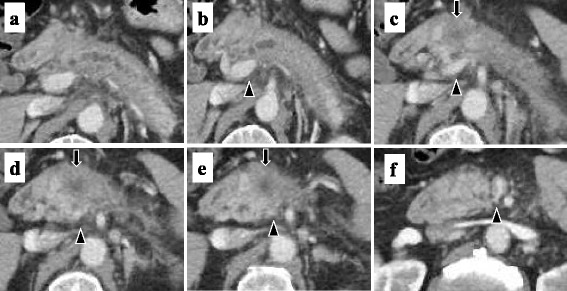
Abdominal contrast-enhanced computed tomography (CT) showed diffuse enlargement of the pancreas with increased CT level in the peri-pancreatic fatty tissue and revealed a mass of 2 cm in size in the pancreas head (c–d, *arrow*). The mass was poorly enhanced in the arterial phase and was gradually enhanced in the venous phase. The portal vein showed narrowing (b–f, *arrowhead*)
